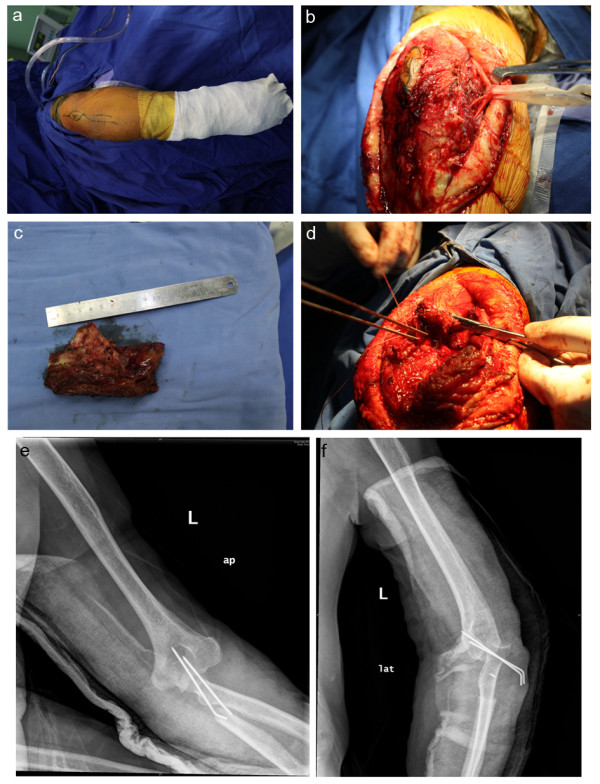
(a) Posterior incision including the biopsy site. (b) Exposure and preservation of the ulnar nerve. (c) Wide excision of the tumor segment. (d) Radius neck-to-humerus trochlear reconstruction; triceps tendon reconstructed to radius head using bone anchor and pins. (e, f) Postoperative radiograph showing cast and pins at 3 weeks.
